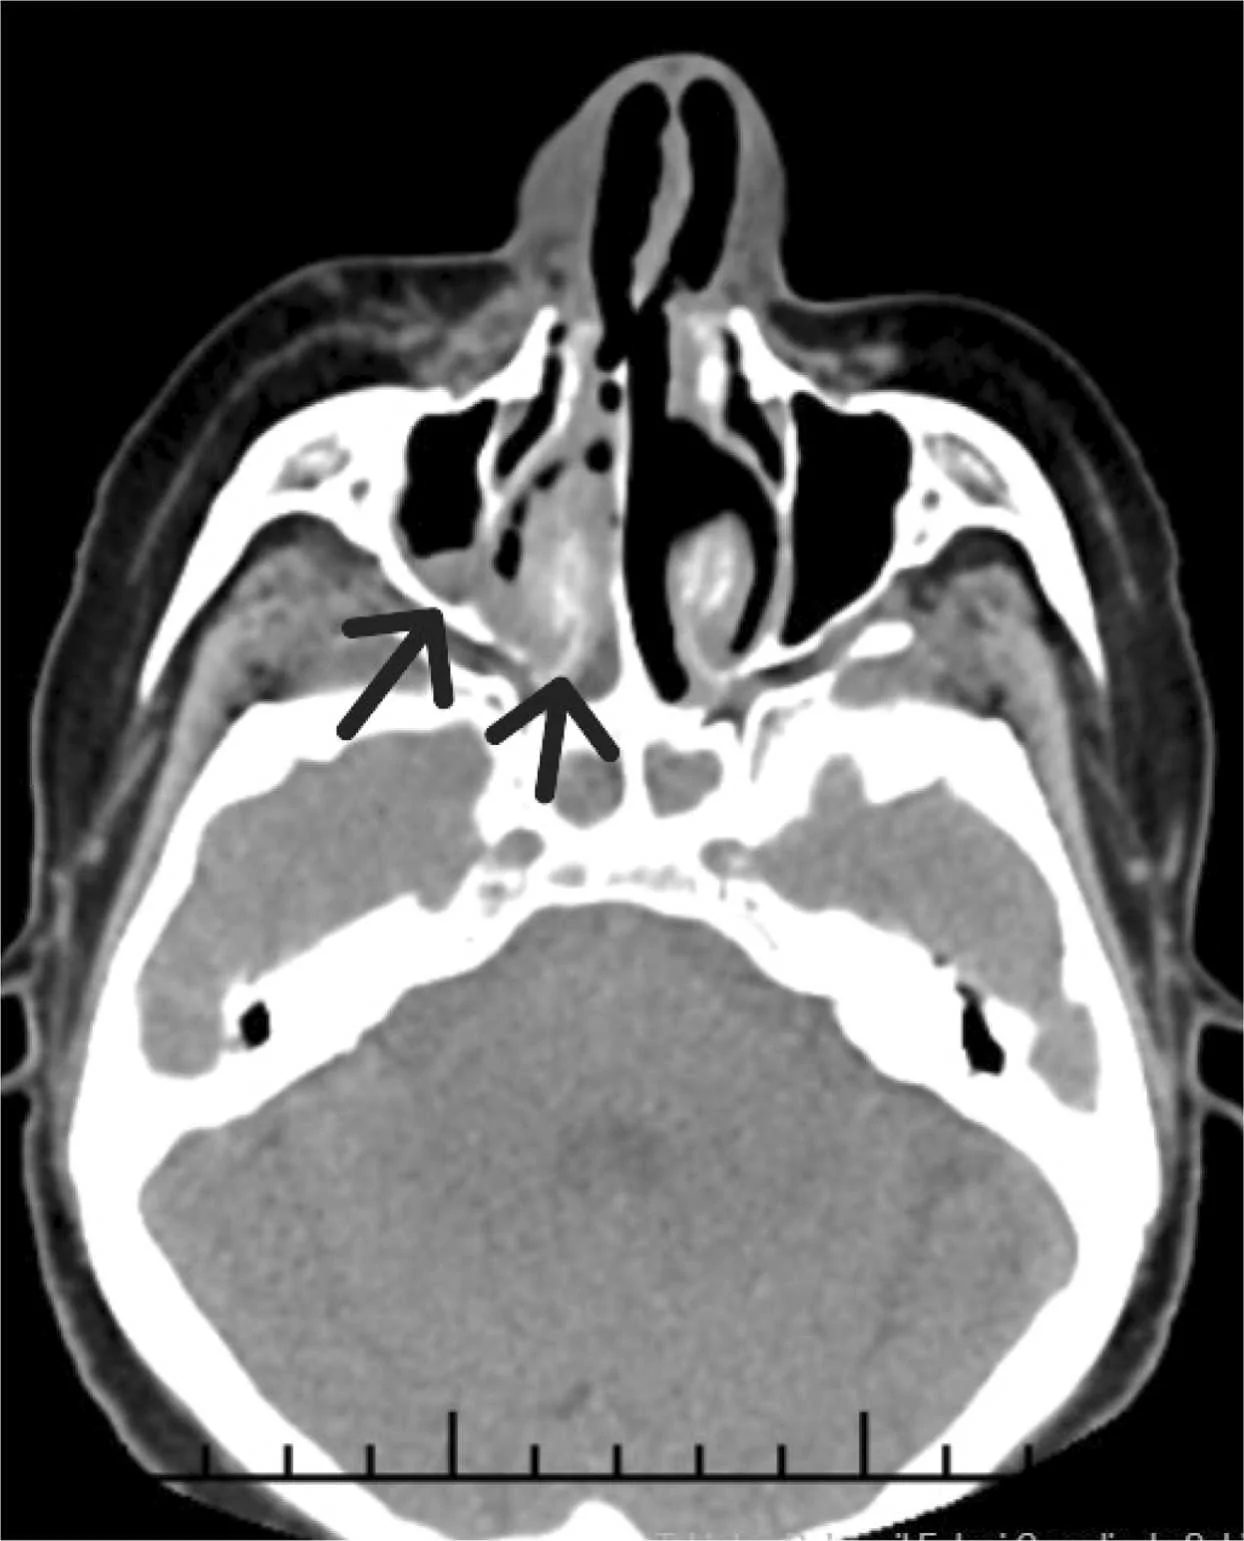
Paranasal sinus CT showing fluid collections and soft-tissue opacification within the right ethmoid and maxillary sinuses.
